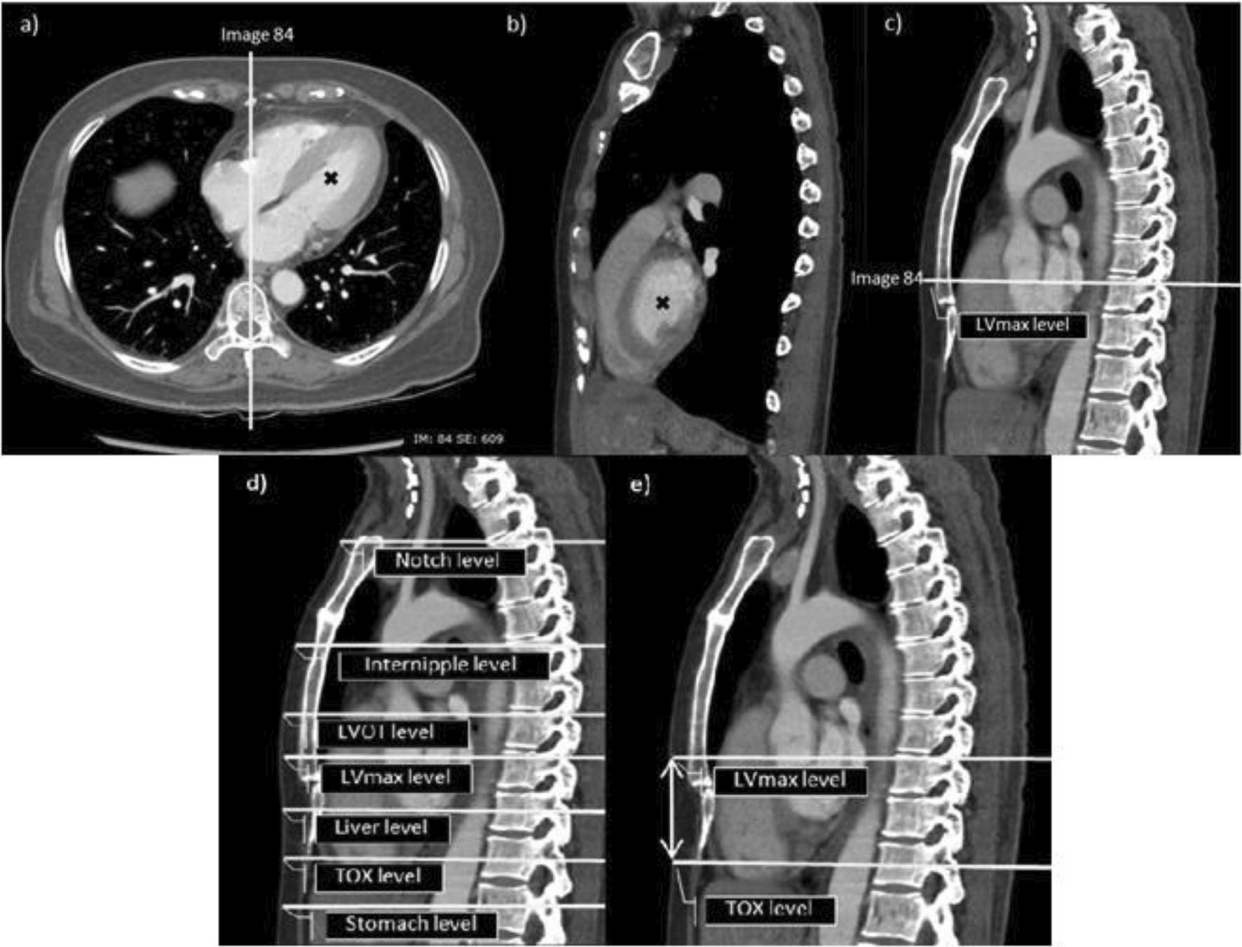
Computed tomography scan demonstrating axial (a), sagittal image at the left ventricular base (b, c) and midline sagittal image (d, e); (a) and (b): The level of maximal width of the left ventricular base (LV_max_) was selected using axial and sagittal images. (a) and (c): The LV_max_ level is located in midline sagittal plane using Interlink function. (d) The level of each reference body landmarks was identified on midline sagittal image and reference lines were drawn. (e) Measurements of the height from tip of xiphoid process to LV_max_ level were done at the points of overlying skin. LV_max_: The level of maximal width of the left ventricular base, LVOT: left ventricular outflow tract, TOX: Tip of xiphoid (Picture by NS. and Patsorn S).
